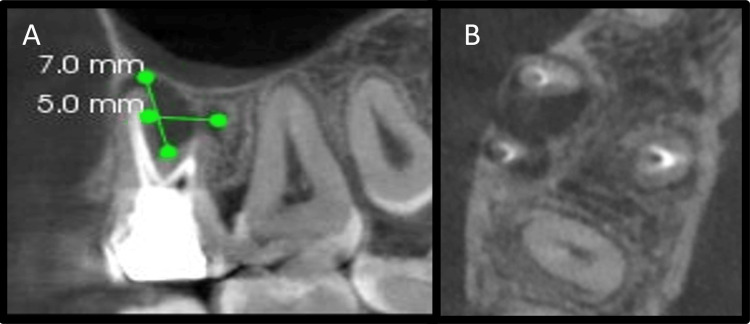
Cone beam computed tomography images A: Cone beam computed tomography sagittal view, B: Cone beam computed tomography axial view
